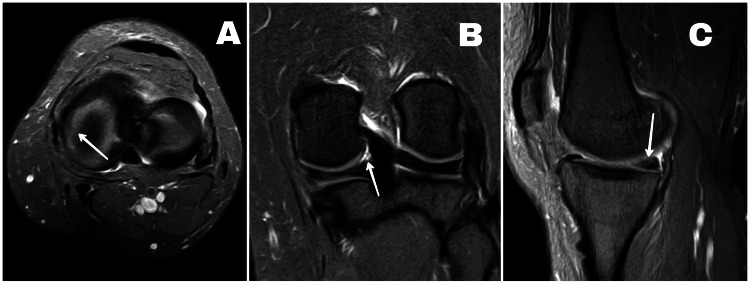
(A) Axial T2-weighted magnetic resonance image of left knee, (B) coronal T2-weighted magnetic resonance image of left knee, and (C) sagittal T2-weighted magnetic resonance image of left knee. The arrow in image A shows a vertical body tear of the medial meniscus, the arrow in image B shows a posterior horn tear of the medial meniscus, while the arrow in image C shows posterior horn tear of the medial meniscus.
